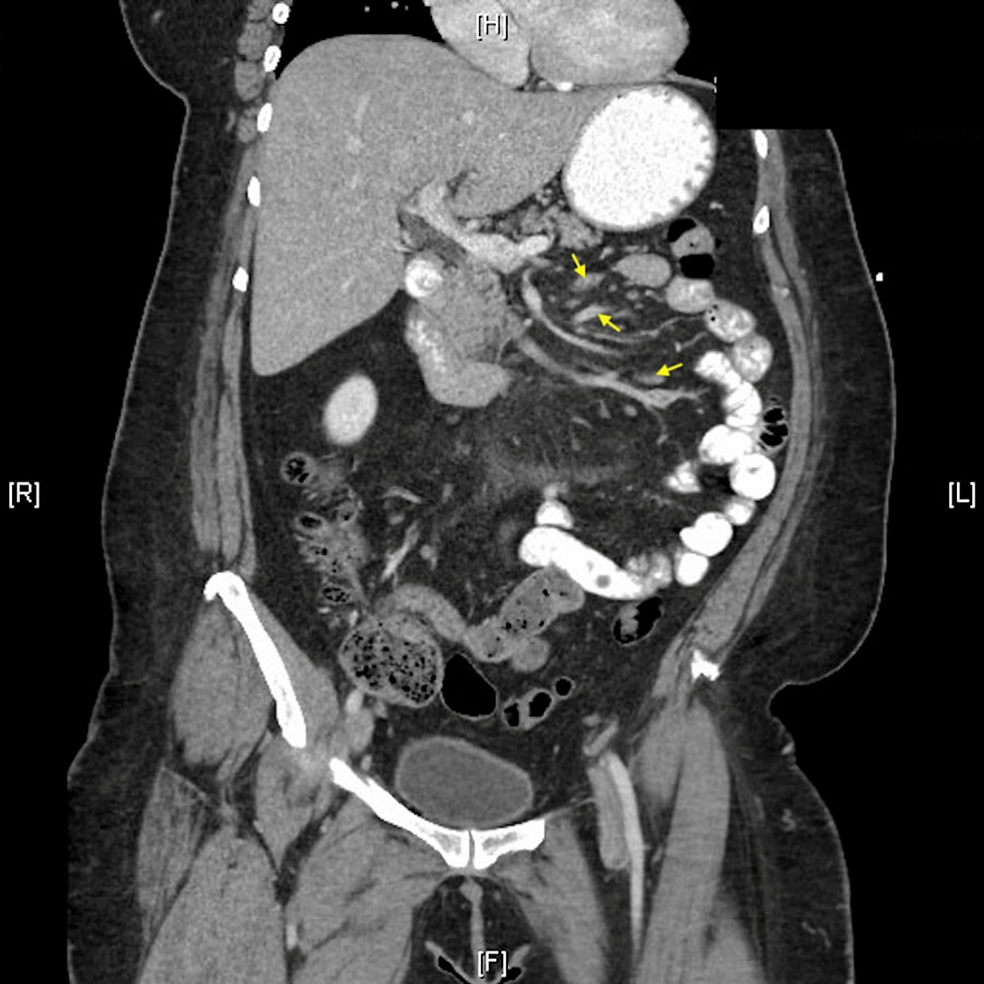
Axial abdominal contrast CT demonstrating mesenteric fat stranding (arrows)
